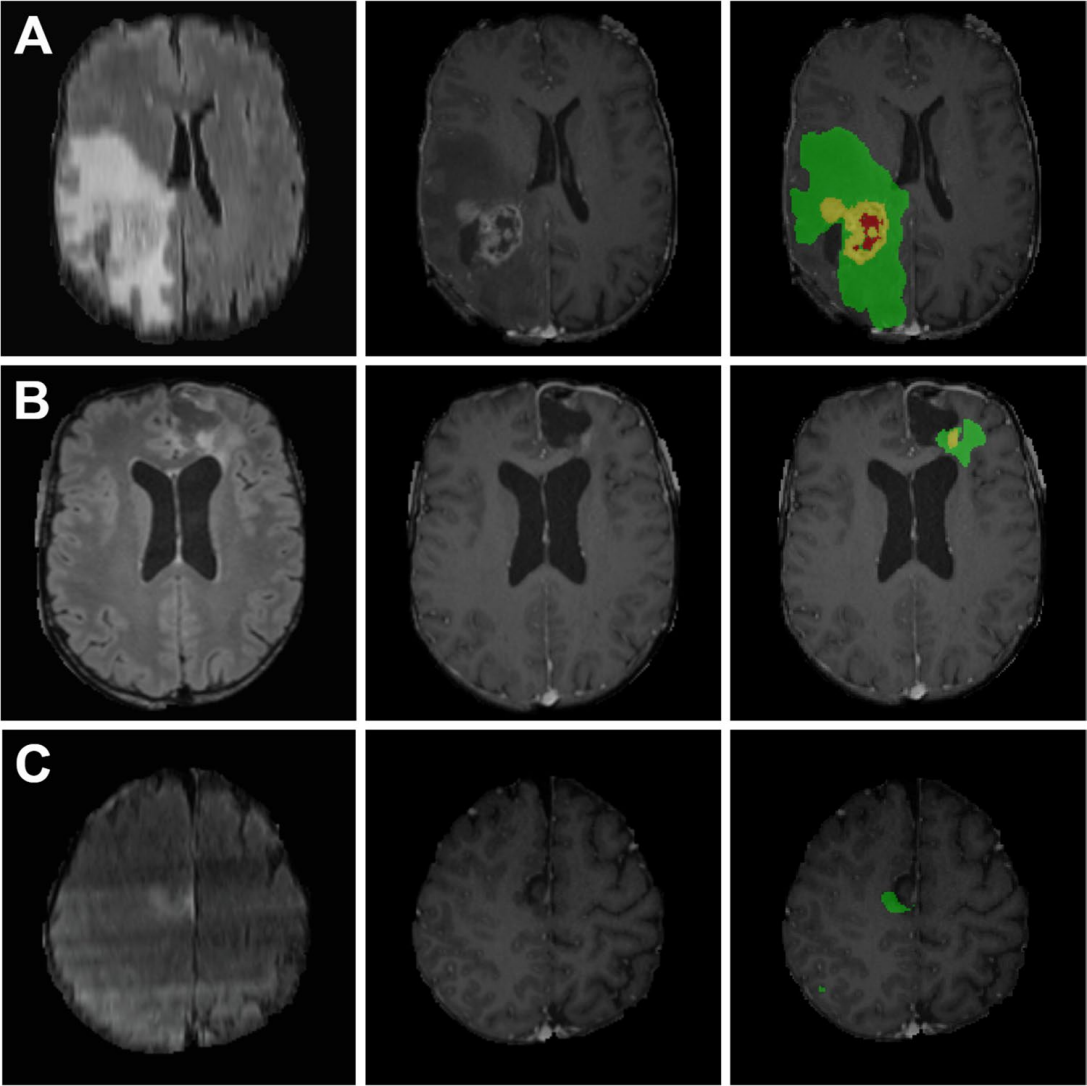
Postoperative holdout set results: Cases were selected as best, median and worst according to *patient wise mean DSC*. Within each row, the skull stripped FLAIR image is shown to the left, the T1 contrast enhanced image in the middle and an overlay with the algorithm generated segmentation to the right side. Edema is displayed in green, enhancing tumor in yellow and necrosis/non-enhancing tumor in red. Metrics are given as DSC: (A) best: ET 0.63, NET 0.50, WT 0.85, mean 0.66; (B) median: ET 0.12, NET 0.00, WT 0.74, mean 0.28; (C) worst: ET 0.0, NET 0.0, WT 0.05, mean 0.02
